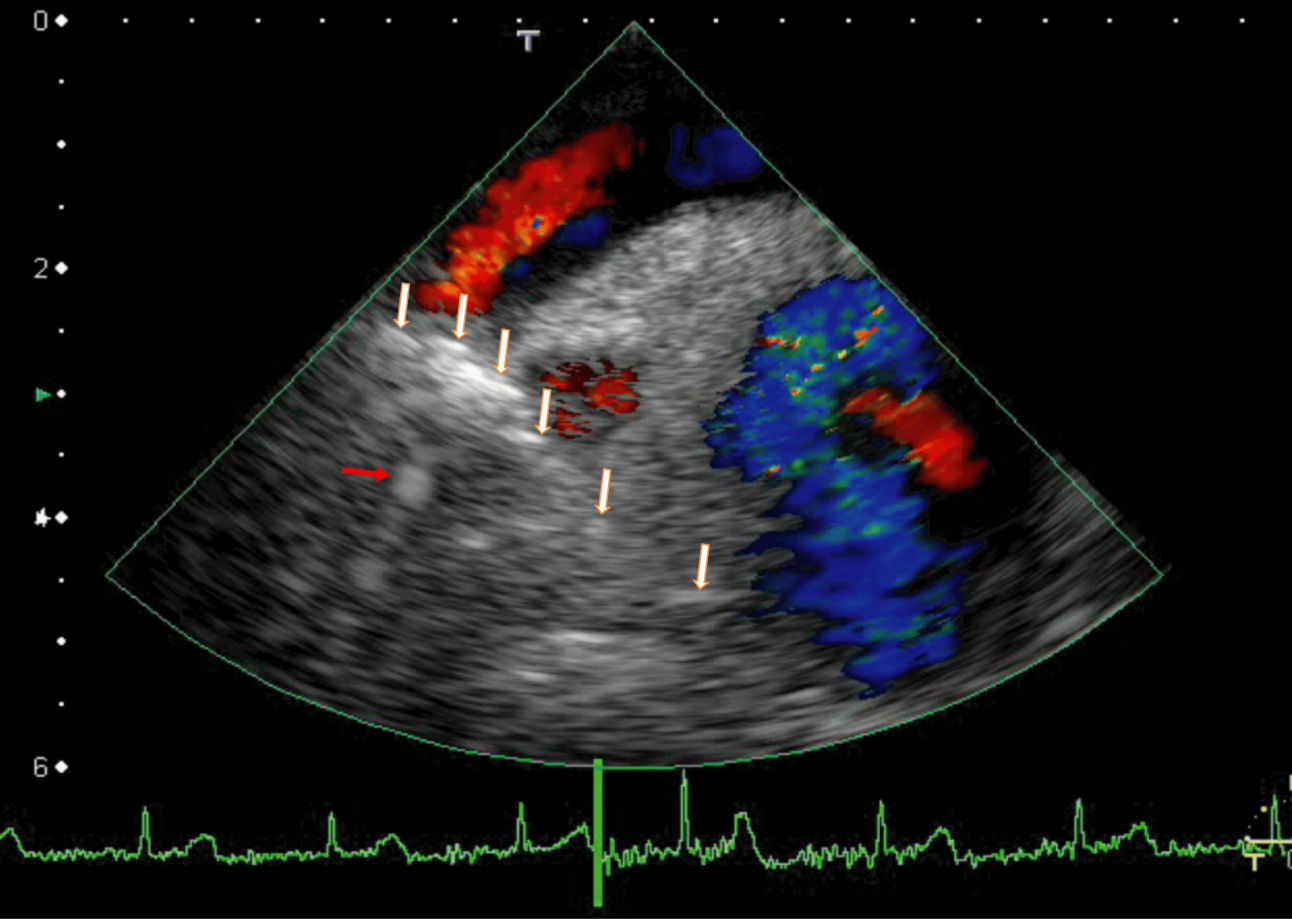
TEE image showing resolution of some vessel thrombi after one year of half-dose apixaban treatment. After one year of half-dose apixaban treatment, TEE revealed that the left-sided vessel thrombi had resolved and did not move with heartbeats. The right-sided thrombi exhibited high-intensity echoes (white arrows). The red arrow indicates a finding with white shadows near the RUPV thrombi. TEE: transesophageal echocardiography; RUPV: right upper pulmonary vein
